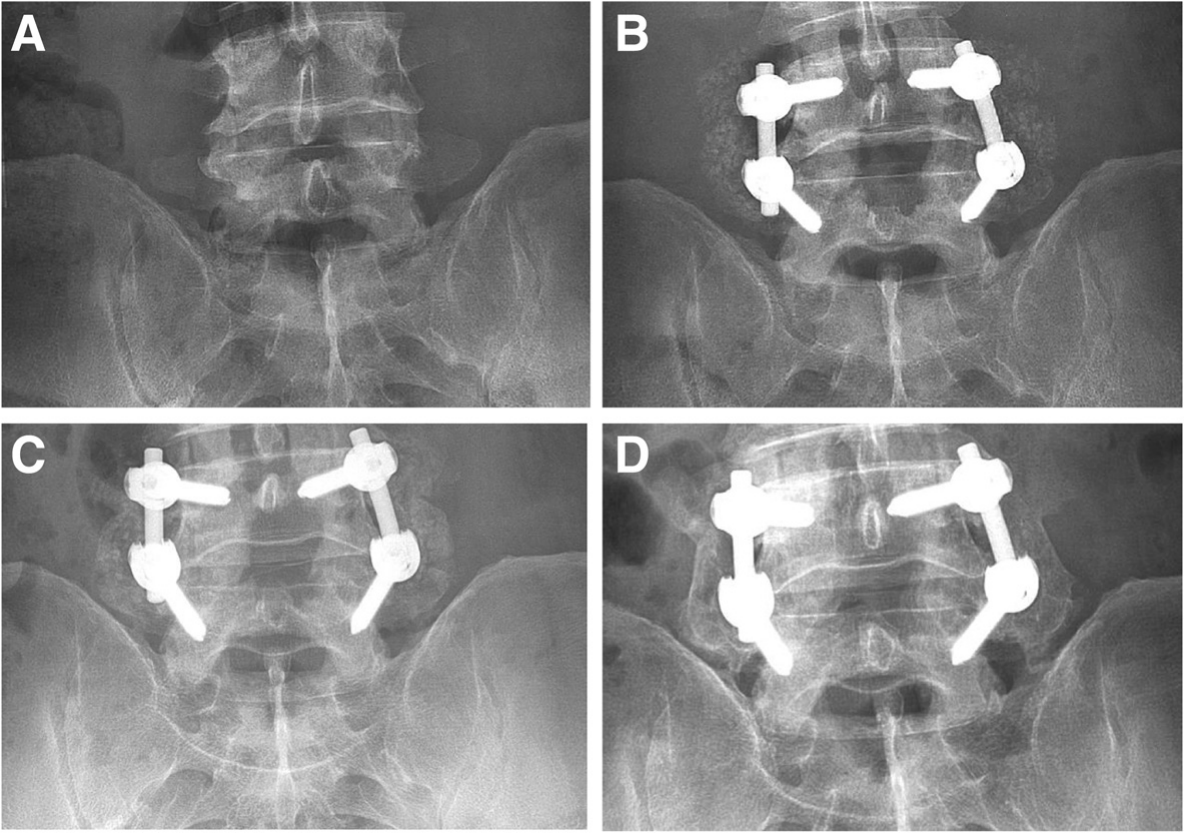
Baseline, 2-week, and 2- and 12-month AP radiographs. These radiographs show maturation of robust bilateral bridging bone over time. Subject was a non-insulin-dependent diabetic patient with grade I degenerative spondylolisthesis. Subject received 10 cc of TE and 15 cc of locally-derived autograft. a Baseline. b 2 weeks. c 2 months. d 12 months
